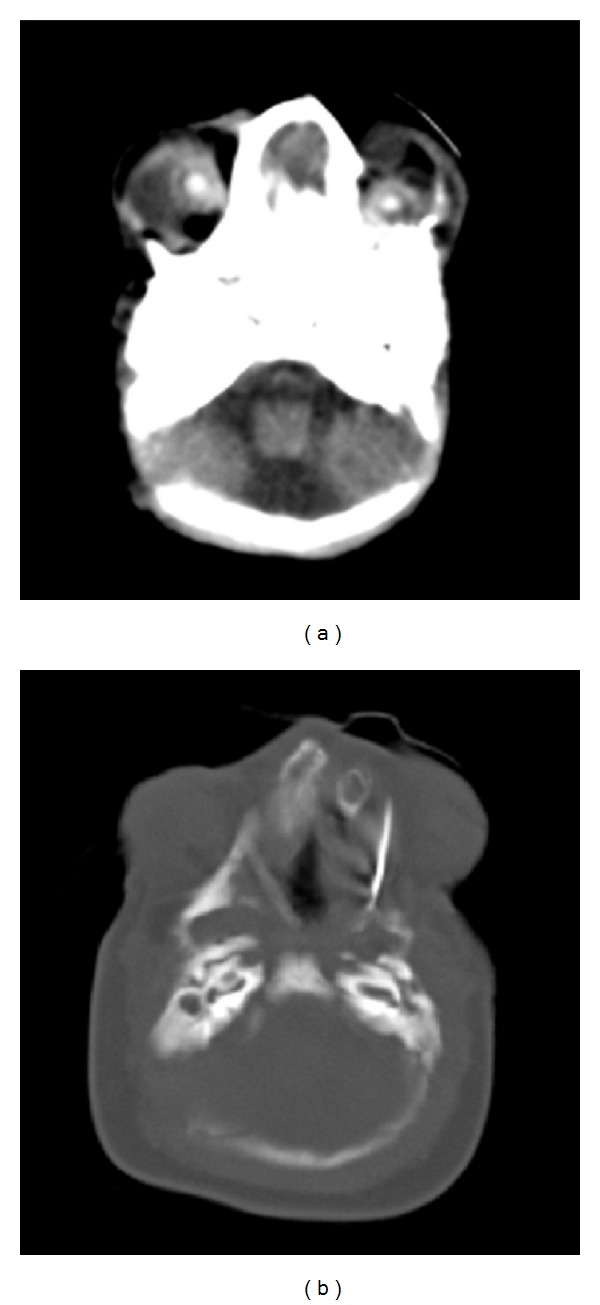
Computed tomography of the cranium, in the axial plane, showing severe hypoplasia of the maxillary bones (a), which were dysmorphic and covered with skin (b).
